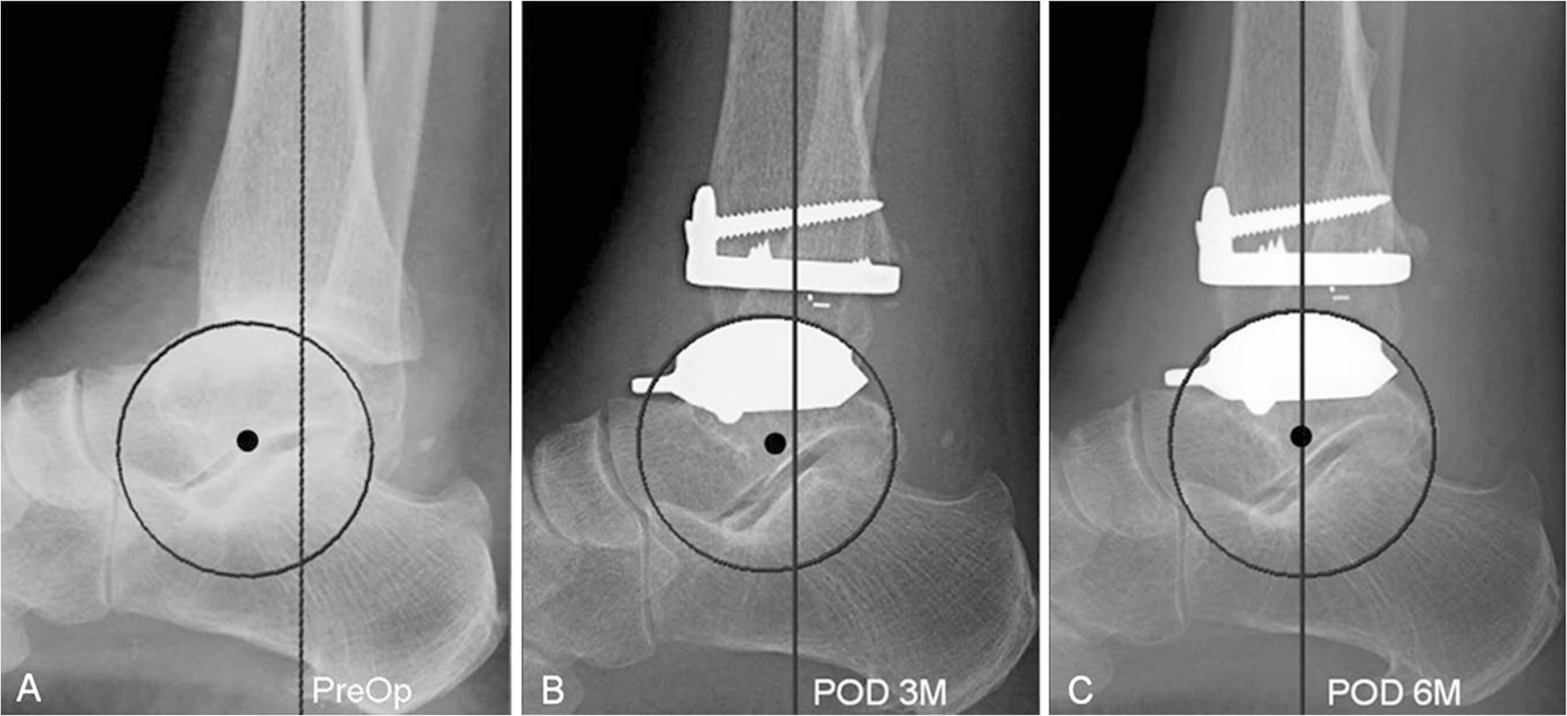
A 42-year-old woman who underwent primary total ankle arthroplasty (A,B,C) for ankle osteoarthritis. (A) Preoperative lateral weight bearing radiograph shows anterior translation of the talus. (B and C) Postoperative lateral weight bearing radiographs show that the anteriorly translated talus was restored to an anatomical position after primary total ankle arthroplasty.
